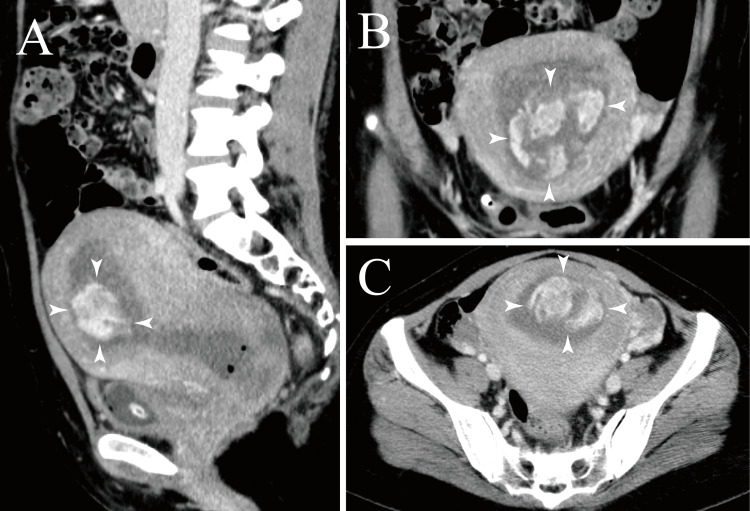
Contrast-enhanced computed tomography image before uterine artery embolization at a regional hospital Computed tomography shows a retained placenta and extravasation (arrowheads) into the uterine cavity. A) Sagittal view; B) Coronal view; C) Axial view
